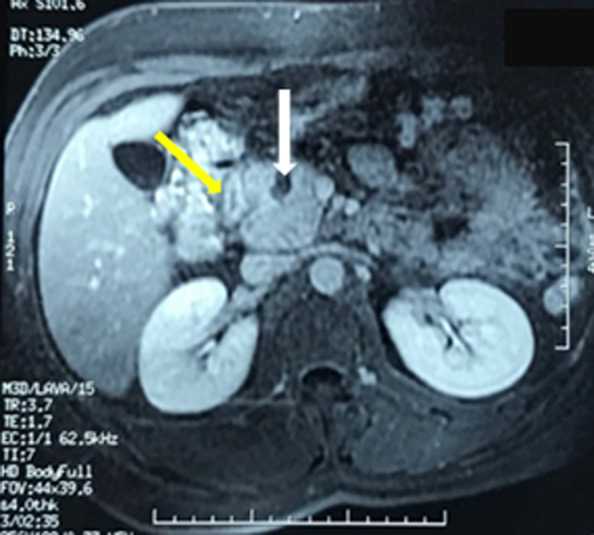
MRI findings: cystic lesions in the head of pancreas (white arrow), a hypertrophic duodenal wall (yellow arrow)
